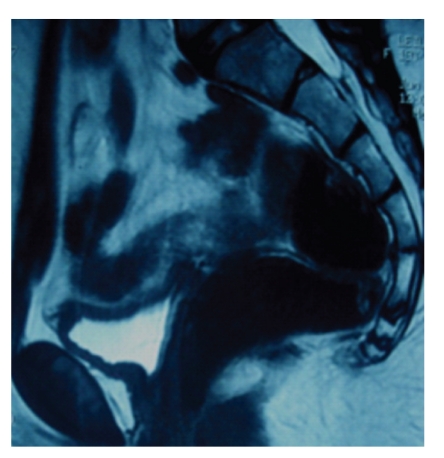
MRI examination sagittal plane cut: absence of uterus and ovaries.
